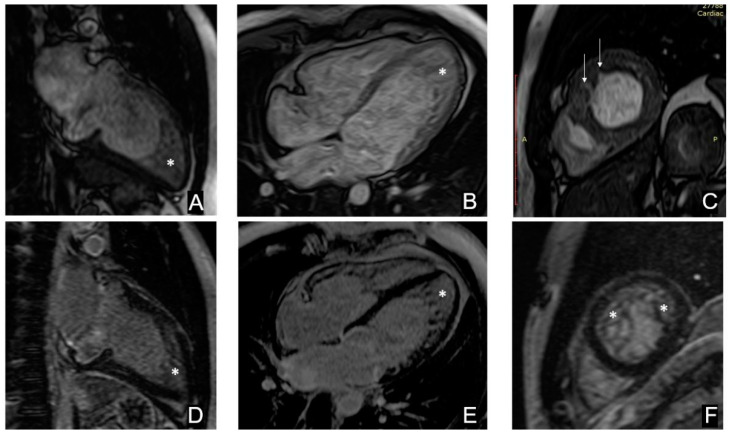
(A–C) The proband’s cardiac magnetic resonance imaging (cMRI) in cine mode, SSFP sequence: (A) long axis 2-chamber images, (B) long axis 4-chamber images, (C) short axis images at the level of the middle segments. (D–F) DE (delayed enhancement) cMRI images, inversion recovery (IR) sequence with suppression of the signal from the normal myocardium. No areas of contrast enhancement were detected, which indicates the absence of areas of intramyocardial fibrosis, scarring or inflammatory myocardial damage. * indicates a layer of non-compact myocardium in the apical segments, → indicates clefts in the middle anteroseptal segment.
